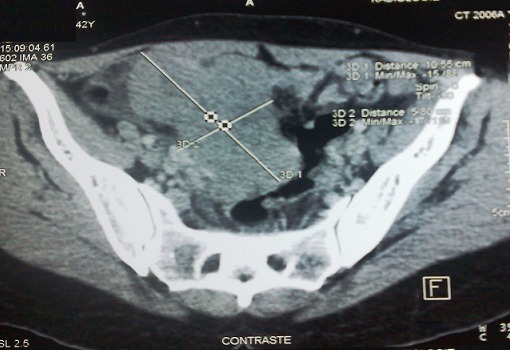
Abdominopelvic CT scan showing a pelvic-abdominal mass with tissue necrosis in places, slightly colored by the injection of contrast
